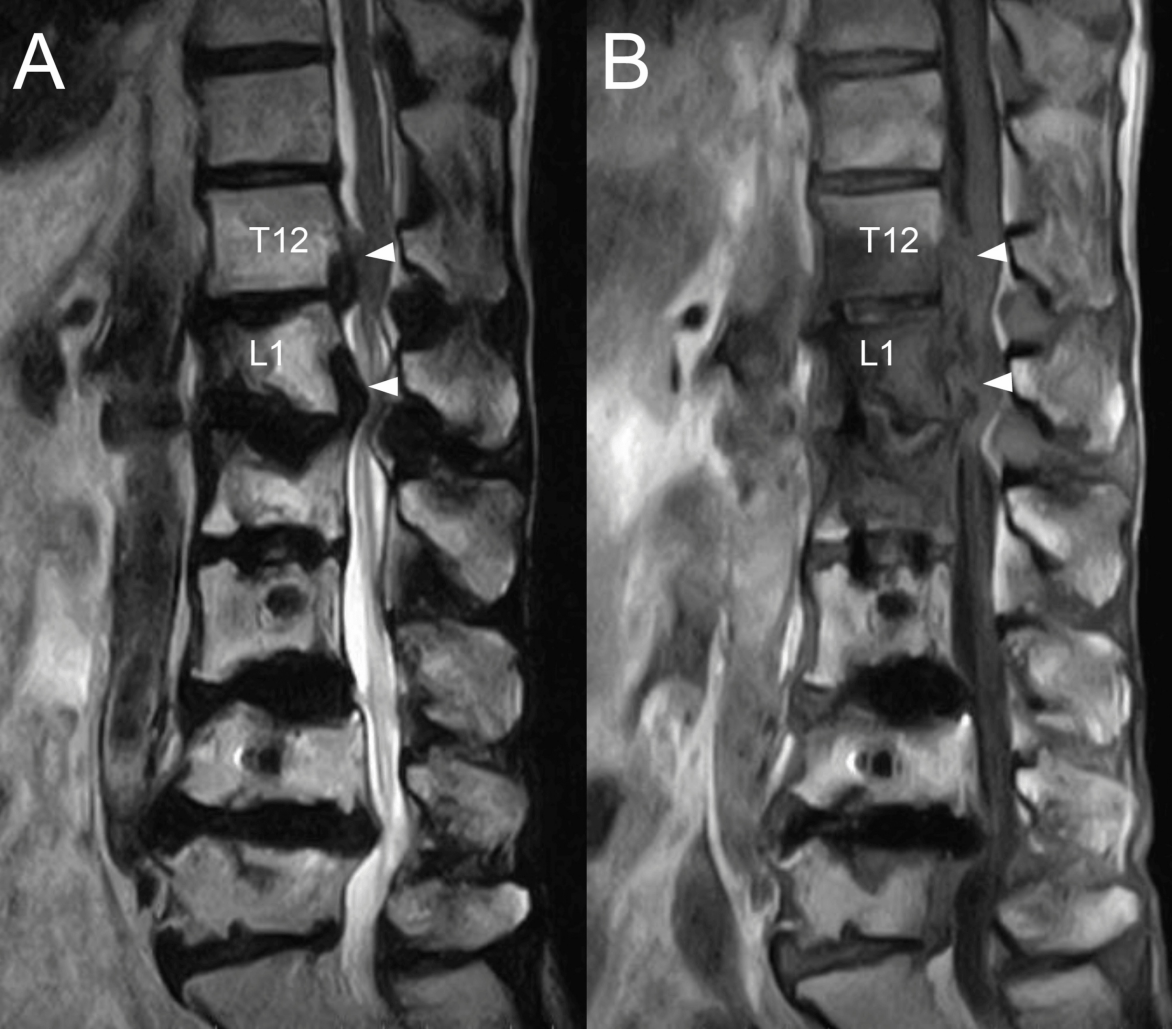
Preoperative MRI images Preoperative MRI images show the epidural lesion (indicated by white arrowhead), which appeared hypointense on T2-weighted images (A) and hypointense to isointense on T1-weighted images (B).
